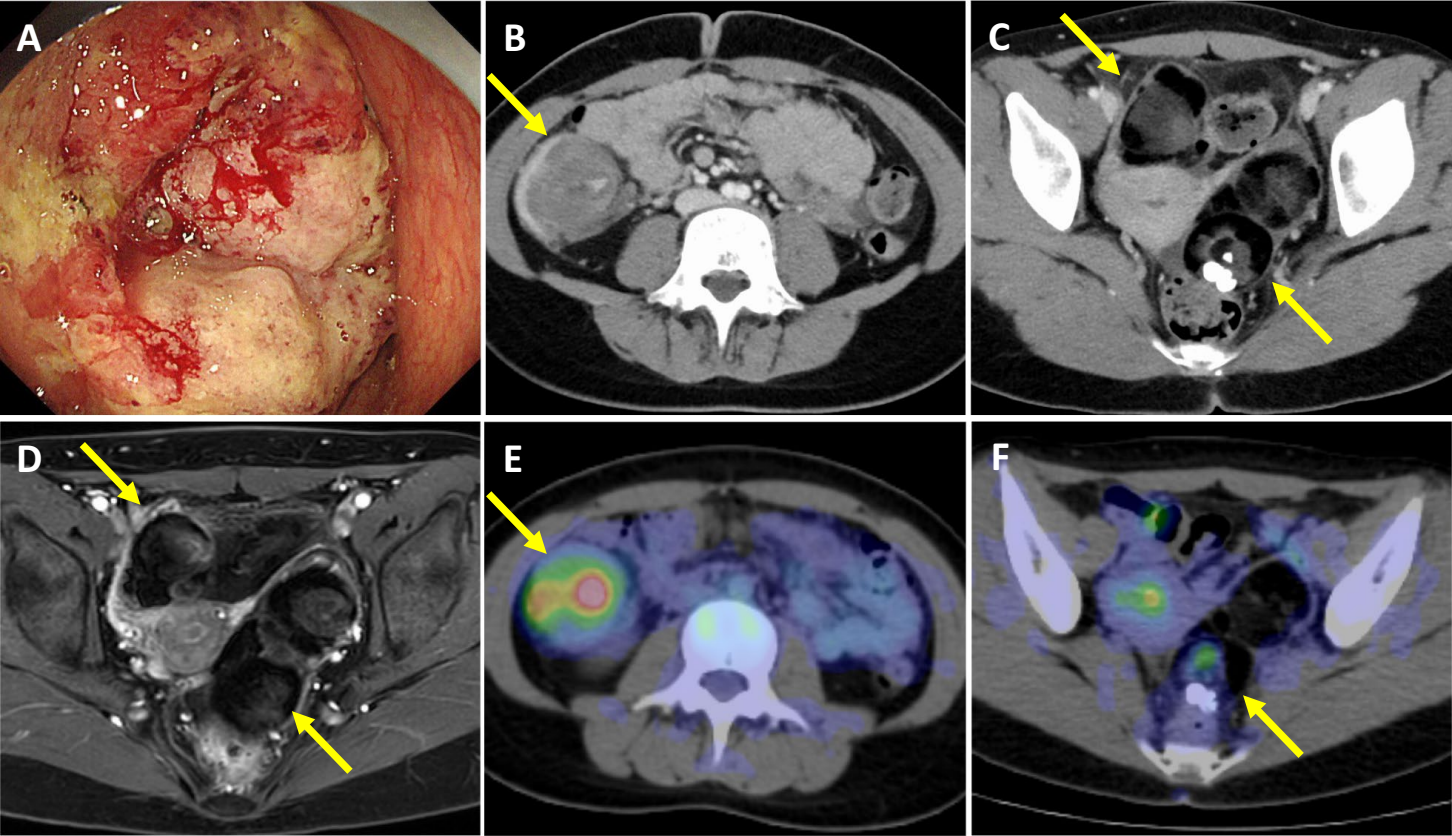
Preoperative images of ascending colon cancer and bilateral ovarian tumors. A Colonoscopy revealed circumferential wall thickening of the ascending colon, which was difficult to pass with the scope. B, C Contrast-enhanced CT showed wall thickening of the ascending colon (B; arrow) and bilateral ovarian tumors (C; arrows). D Pelvic MRI showed bilateral ovarian tumors (arrows). E, F FDG–PET showed the increased uptake of FDG at the ascending colon (E; arrow) and bilateral ovarian tumors (F; arrows)
